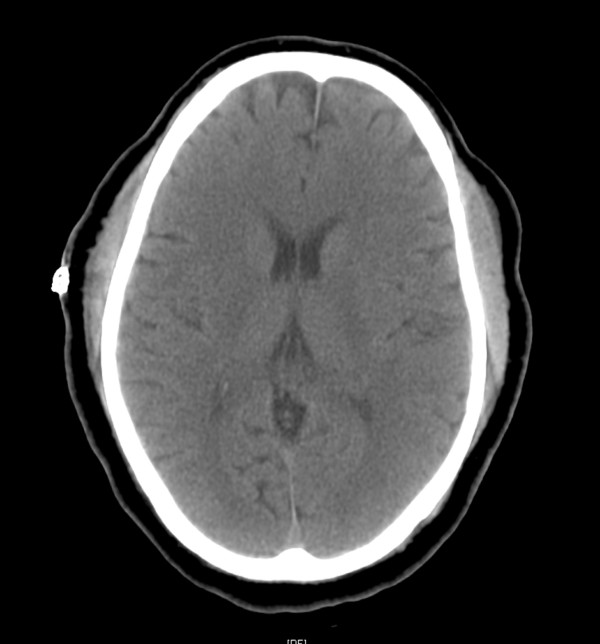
Post-operative axial CT of head showing no intracranial pathology.
